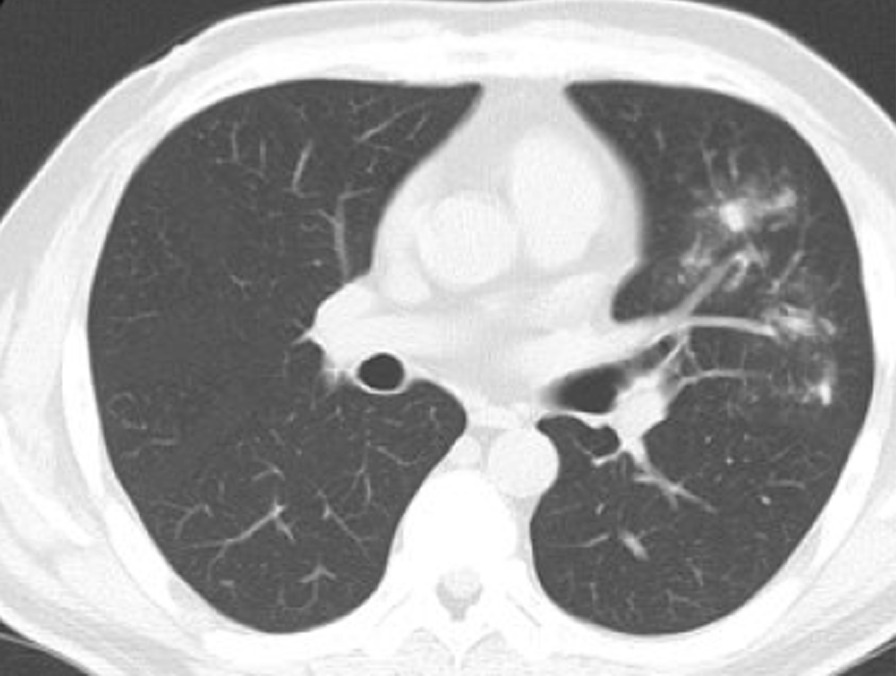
Chest computed tomography after PSL administration (10 mg/day). At 41 days after the administration of increased doses of PSL (when the PSL dose reached 10 mg/day), chest computed tomography revealed infiltrative and granular shadows in the left lung. *PSL* prednisolone
